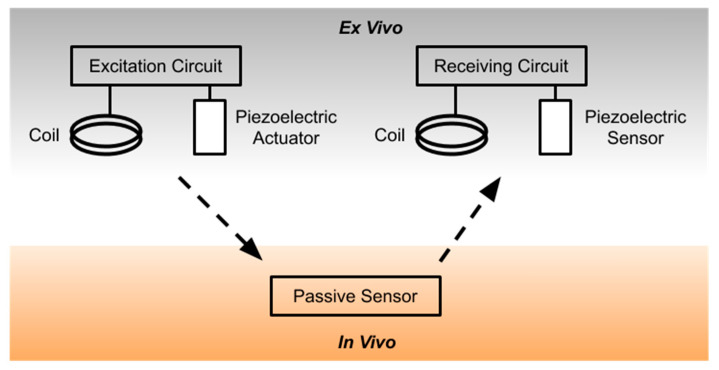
The design of a generic passive interrogator. A signal is generated in the excitation circuit and transmitted at radiofrequency (RF) using an inductive coil or at ultrasound frequencies using a piezoelectric element. The reflected signal is received by a receiving circuit using a matching coil or piezoelectric element.
